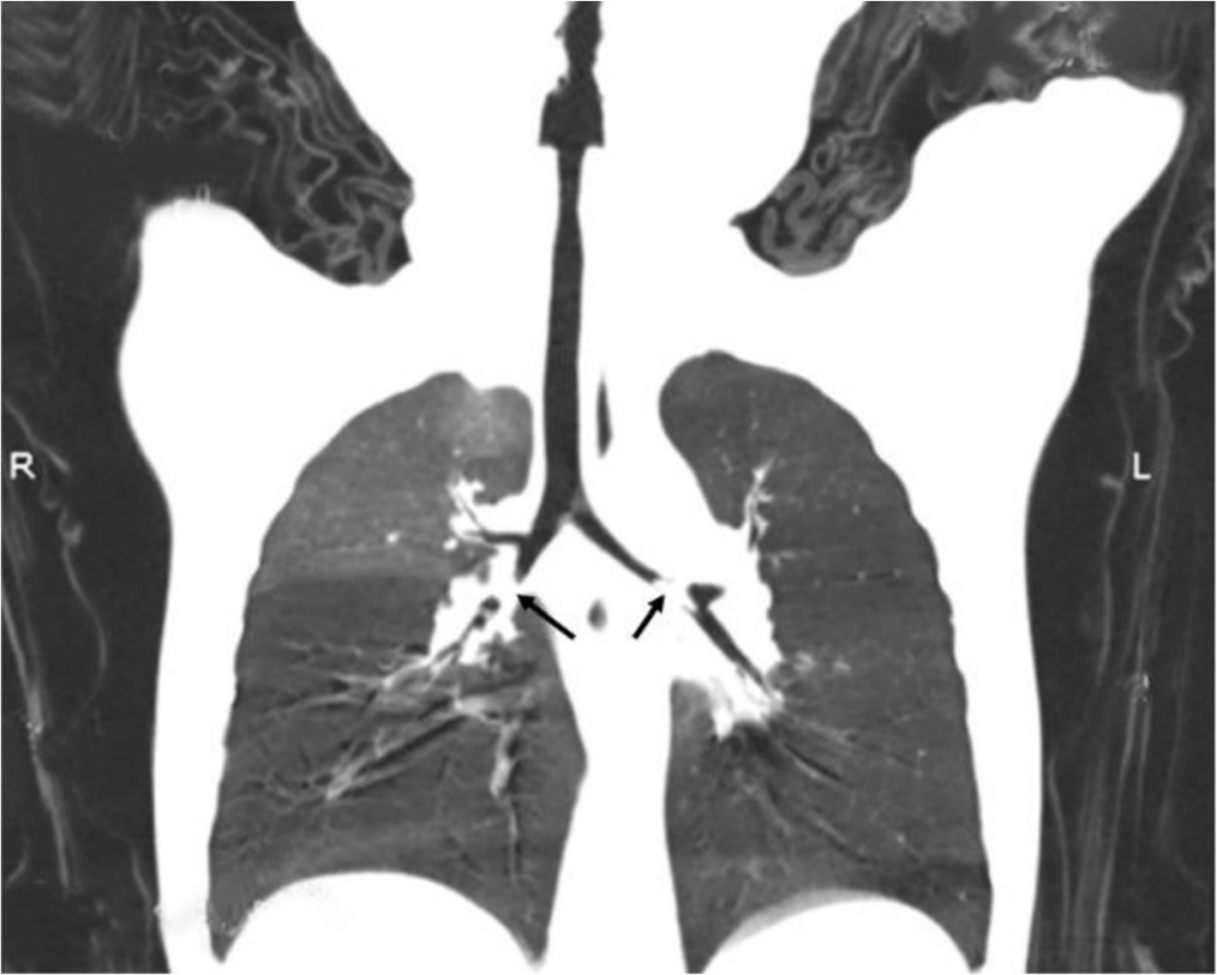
Multislice spiral CT showed a bilateral bronchial foreign body (peanut)
